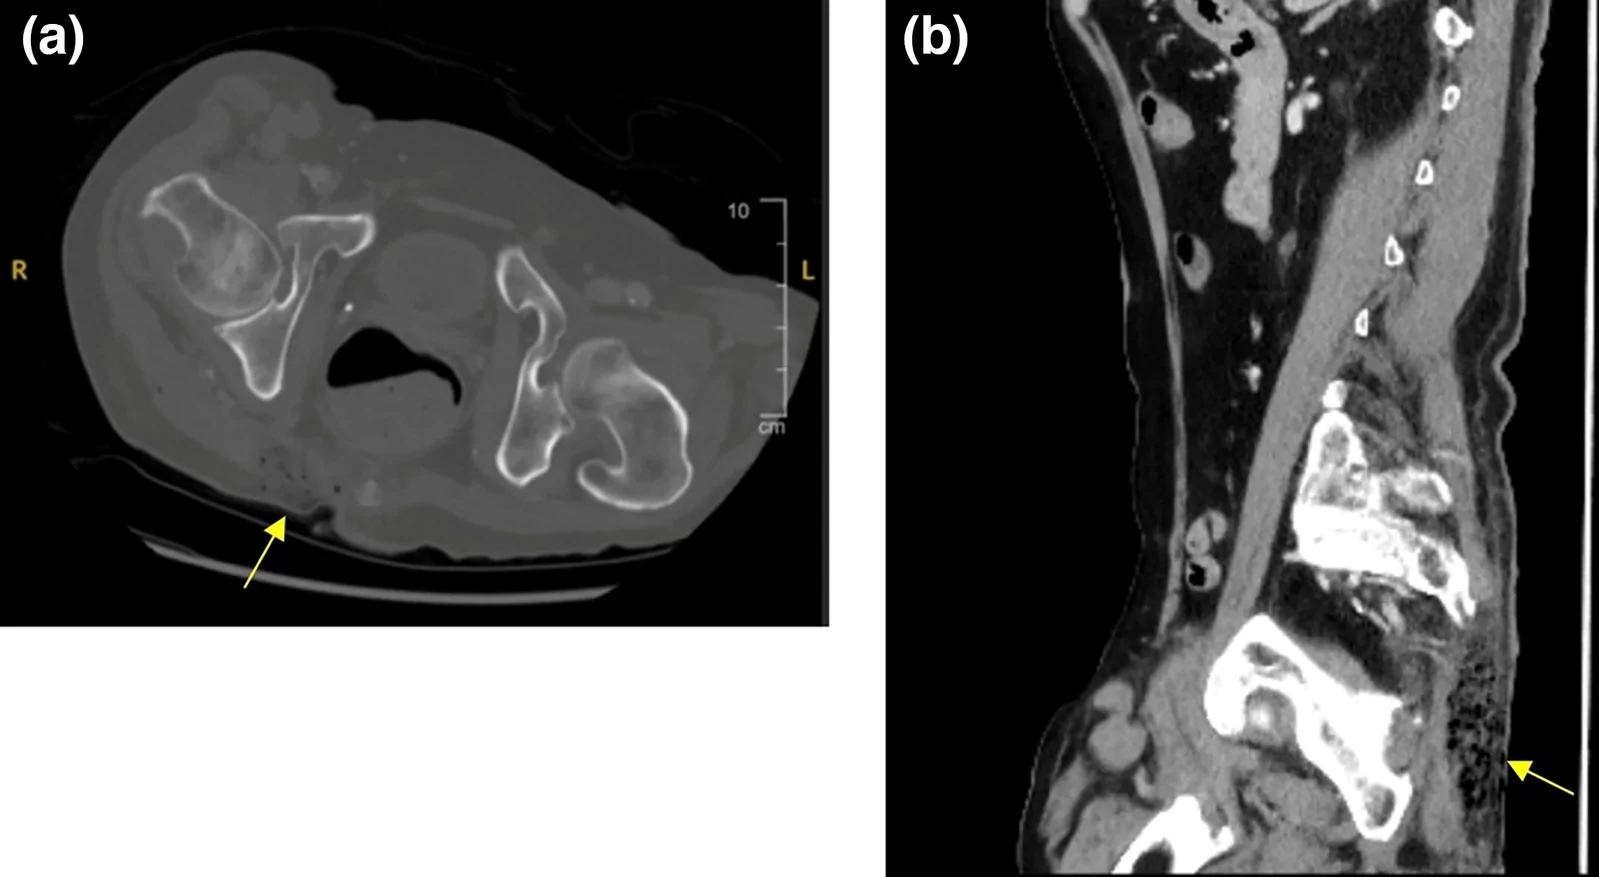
(a) Axial and (b) Sagittal cuts on the patient’s CT scan showing a large right sacral wound with surrounding inflammatory changes as well as gas locules and tracks in the soft tissue.
